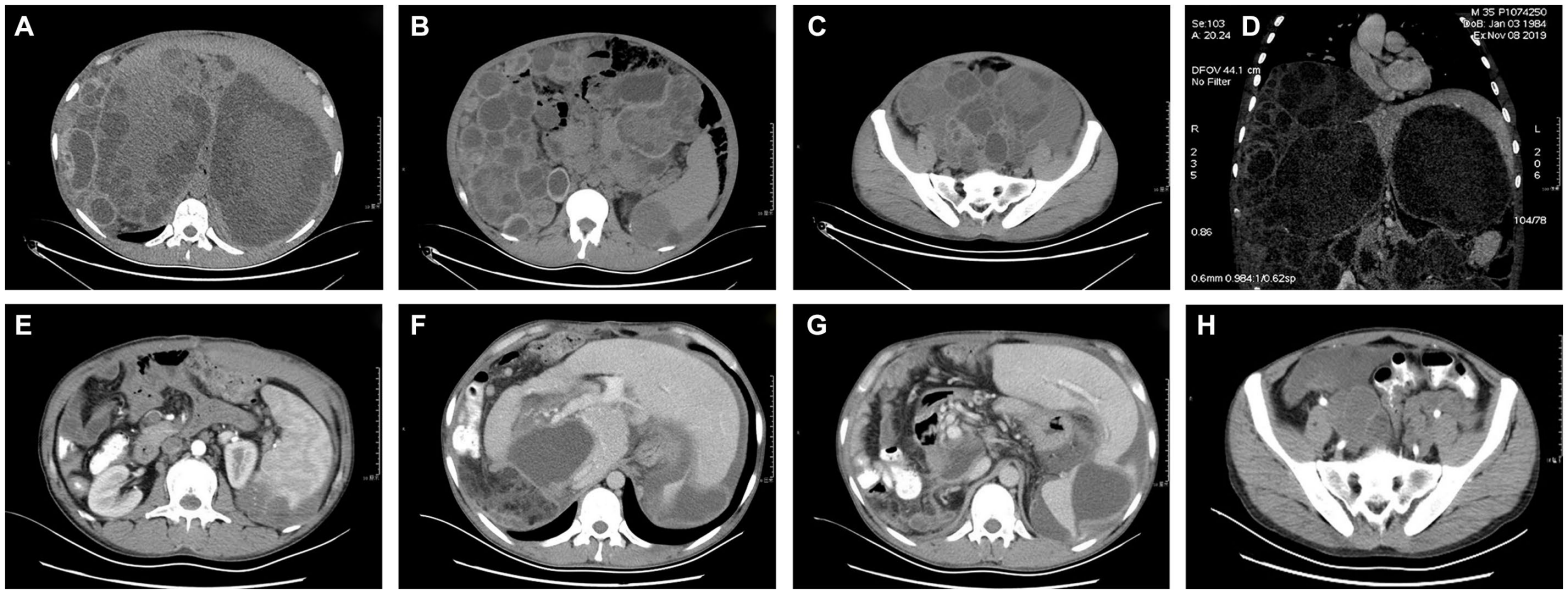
(A-D) Preoperative CT images of the pelvis and abdomen. (E-H) Postoperative CT images of the pelvis and abdomen.
